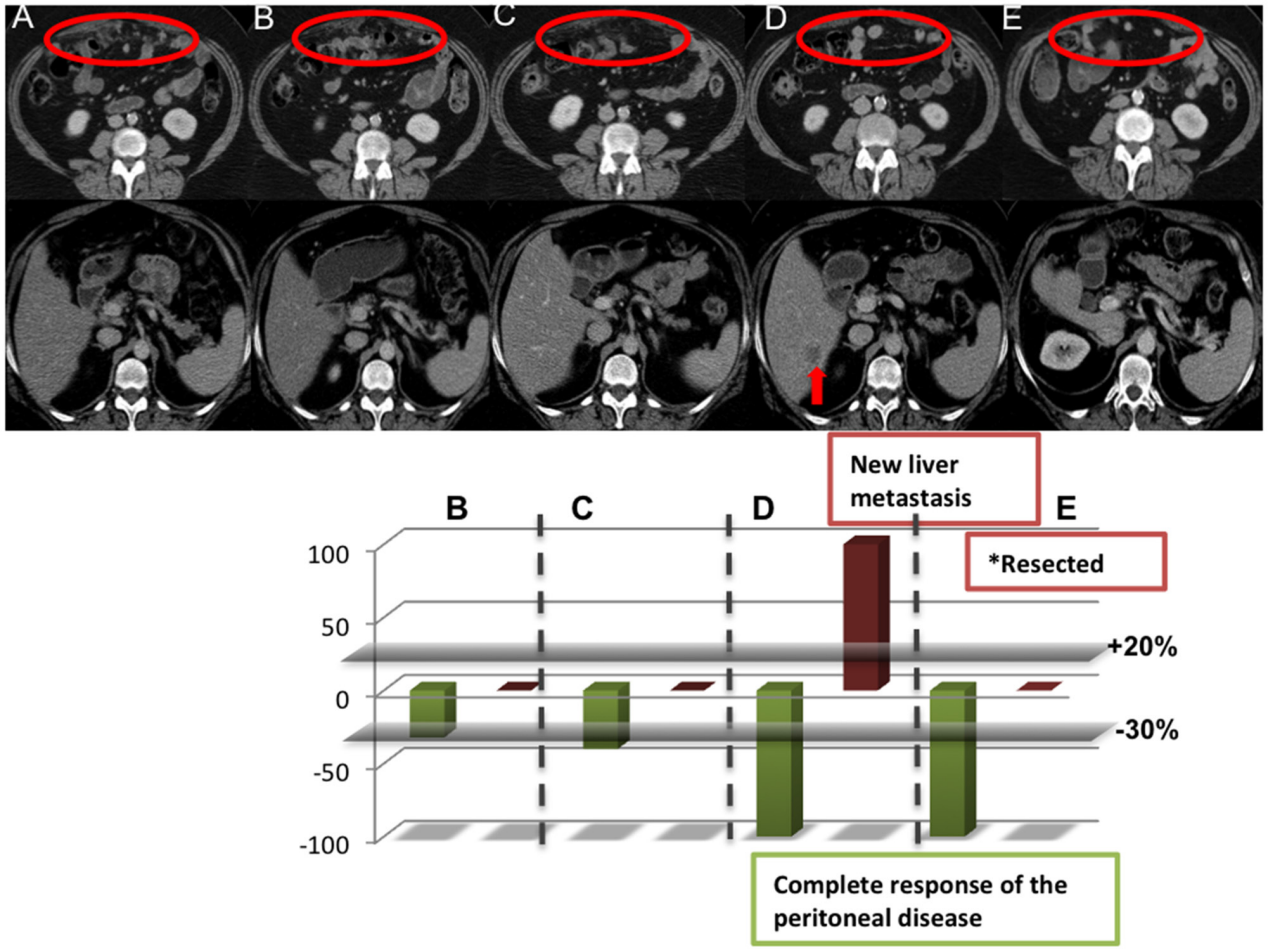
Tumor characterization of a PARP inhibitor responder with RDR Axial enhanced CT images in a 52-year-old female with germline BRCA2 mutation papillary serous ovarian carcinoma who commenced PARP inhibitor monotherapy within a phase I clinical trial in July 2007. (A) The baseline CT shows multiple peritoneal deposits measuring up to 25mm (circle) and no metastatic liver disease. (B) The 12 weeks and (C) 24 weeks CT show marked reduction in size of the peritoneal deposits (circle) and no liver metastases. (D) A subsequent CT in November 2013 showed an excellent response in the peritoneal disease with no CT evidence of peritoneal disease relapse or ascites but, with a new solitary liver metastasis in segment VI (arrow), which was subsequently surgically resected. (E) A subsequent CT in June 2015 shows maintained complete radiological response.
